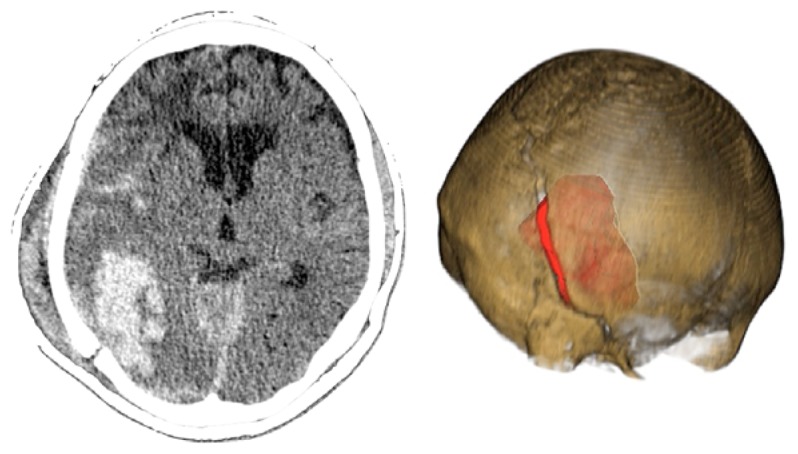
CASE 3. Preoperative CT scans. The 3-D environment with transparency control enables the surgeon a faster understanding of the needs and limitations of this case. Furthermore, it is possible to calculate the volume of hematoma more accurately, which helps guide the percent of hematoma was resected during surgery. In this case we prefer to avoid the site of suture disjunction.
